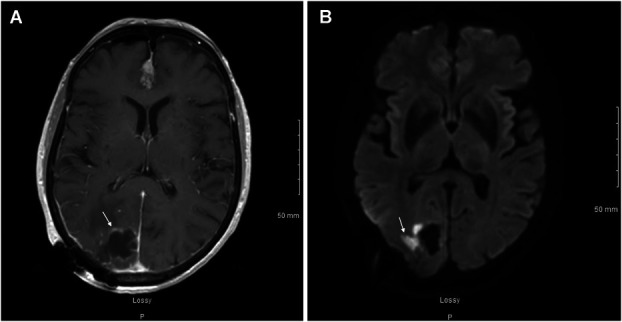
Axial T1 MRI postcontrast A, postresection showing the postoperative cystic cavity demonstrating some mild peripheral enhancement and a small, lateral, nodular area of enhancement (white arrow), as well as a postresection axial diffusion-weighted imaging B, demonstrating small areas of diffusion restriction on the lateral side of the cavity, largest measuring up to 1.5 cm (white arrow).
